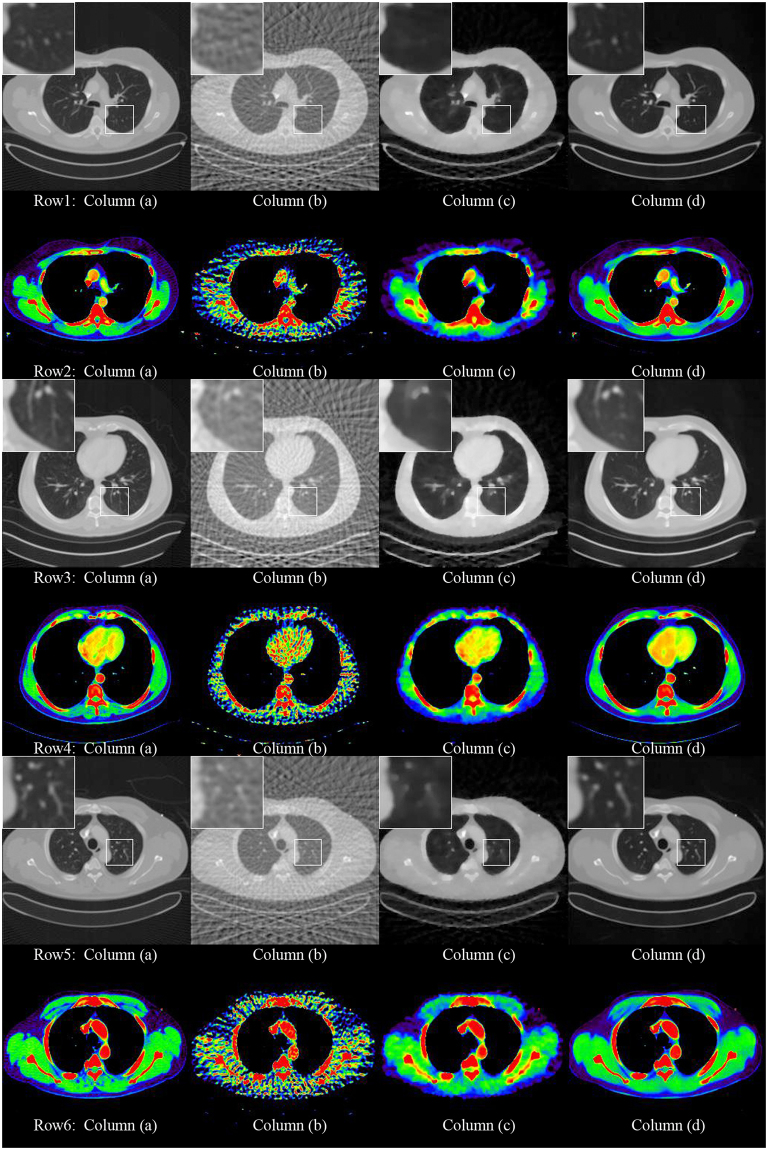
The 512*512 CT images reconstructed by 60 projection views. Column (a) shows the reconstruction by the FBP method from the full projection views. Column (b) shows the reconstruction by the FBP method from the sparse projection views. Column (c) shows the reconstruction by the ADS-POCS method from the sparse projection views. Column (d) shows the reconstruction by the proposed method. All images display in the same window at row 1, 3 and 5. In order to compare the CT value with different reconstruction method, we use 2D atlas to display. The results are shown in row 2, 4 and 6.
